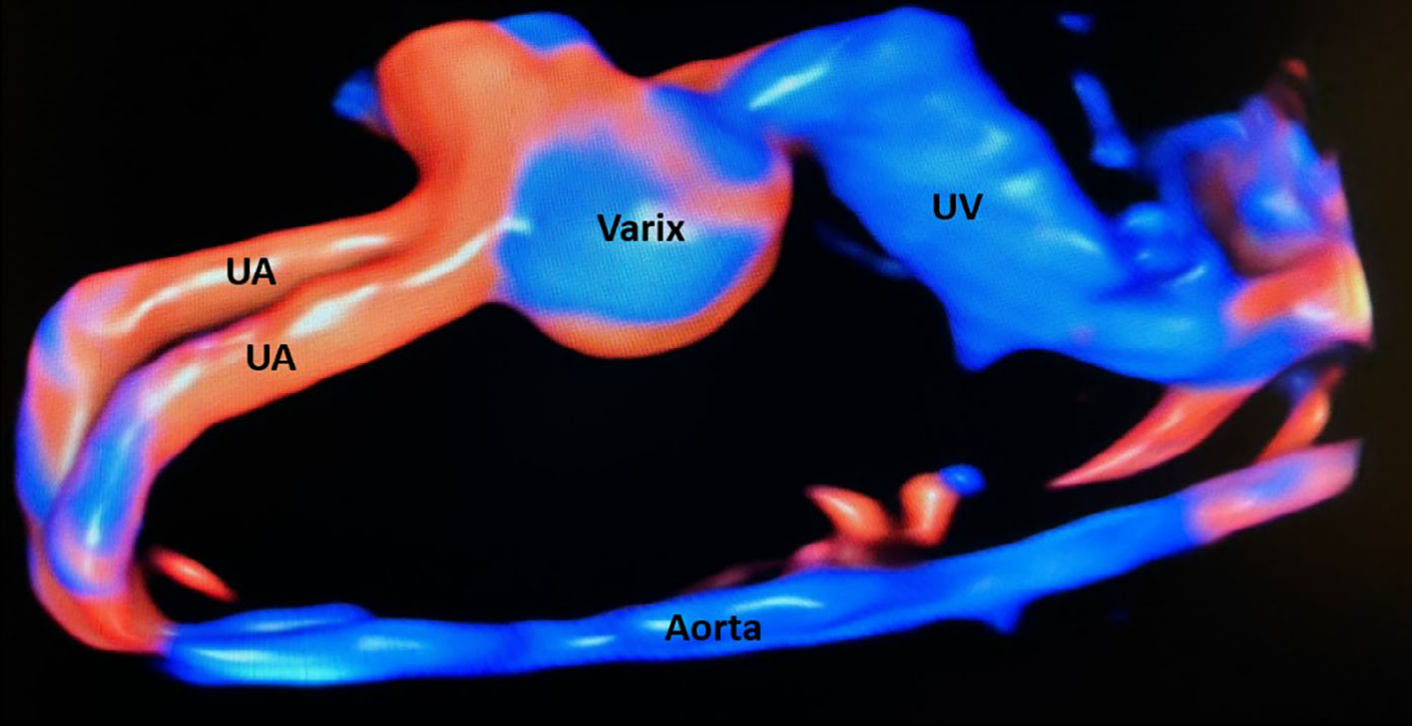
Sagittal view of the fetal abdominal vascular system demonstrating a varix in the umbilical vein on 3D ultrasound. UA, umbilical artery; UV, umbilical vein.
